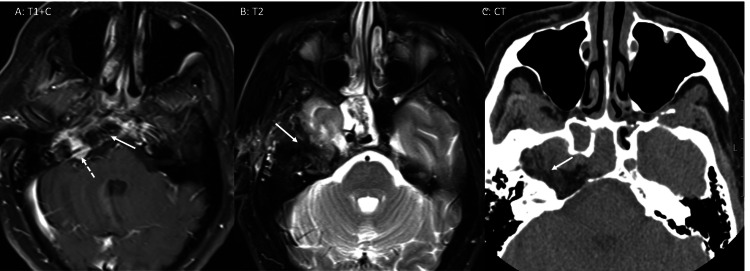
Follow-up MRI after resection of the mass Follow-up MRI after resection of the mass shows post-operative changes due to the presence of a fat graft (arrows in A, B, and C). A small residual enhancing lesion is present (dashed arrow in A) in the jugular foramen.
